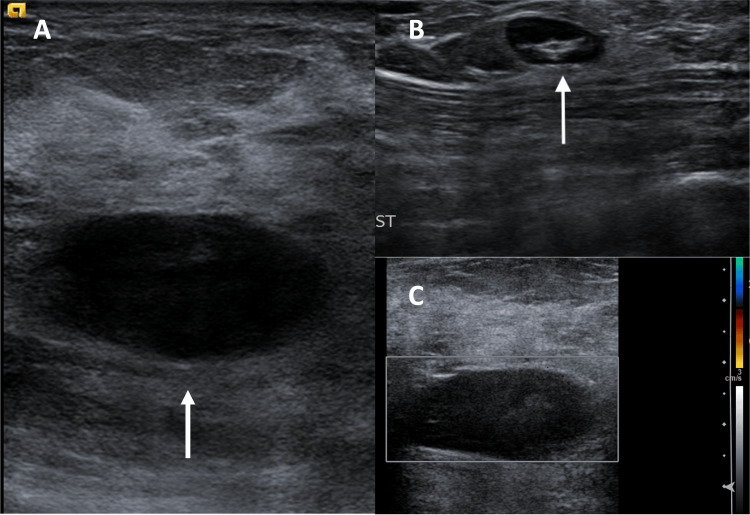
(A) Transverse ultrasound of the left breast demonstrates a 3.5 x 1.5 x 3.0 cm hypoechoic oval mass at the 8 o’clock position, 4 cm from the nipple. (B) Ultrasound of the left axilla demonstrates a 1.0 cm lymph node with mild cortical thickening. The axillary lymph node was determined to be benign with no identifiable malignancy according to pathology. (C) Transverse ultrasound with color Doppler showing no color vascular flow.
